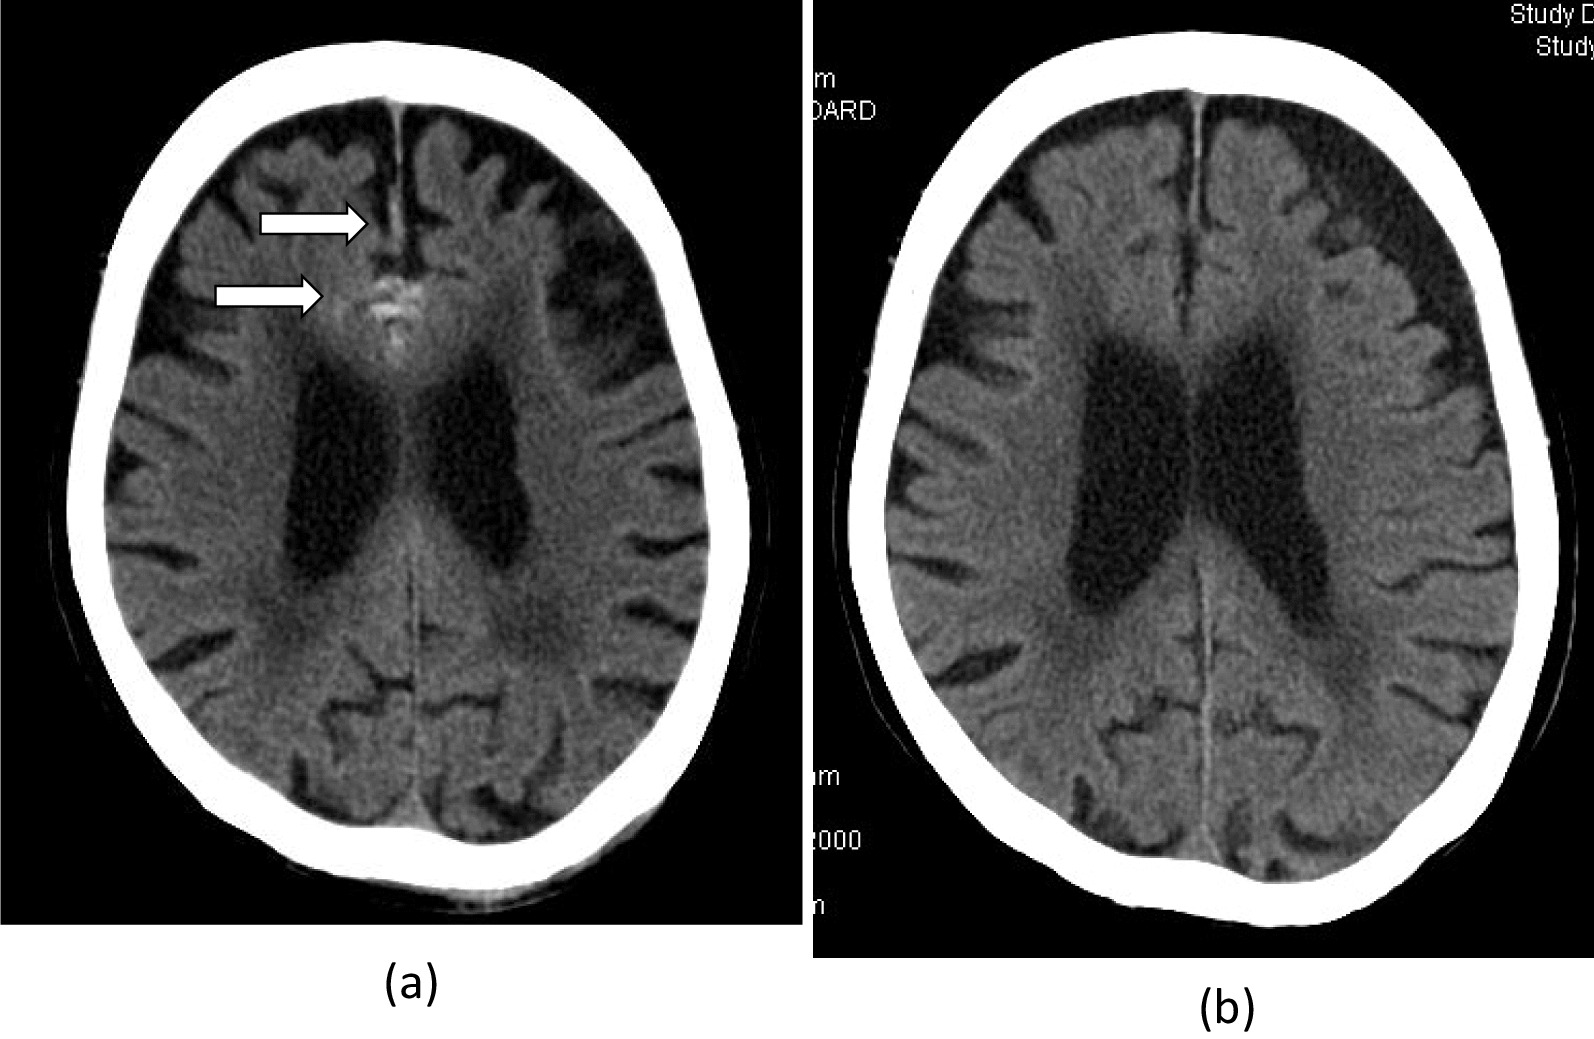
a Brain computed tomography (CT) on admission showing subarachnoid and subdural hemorrhages (arrows). b Repeat brain CT showing the disappearance of the subdural hemorrhage
